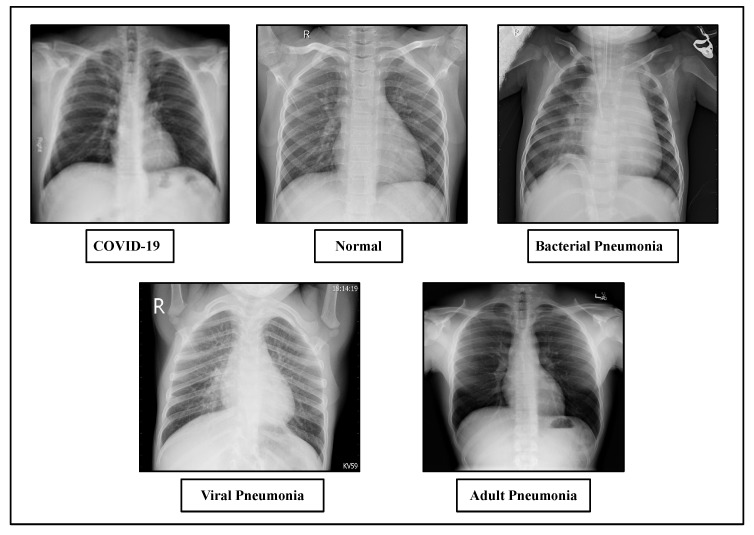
Sample chest X-ray of COVID-19, normal, viral pneumonia, bacterial pneumonia and adult pneumonia cases.
